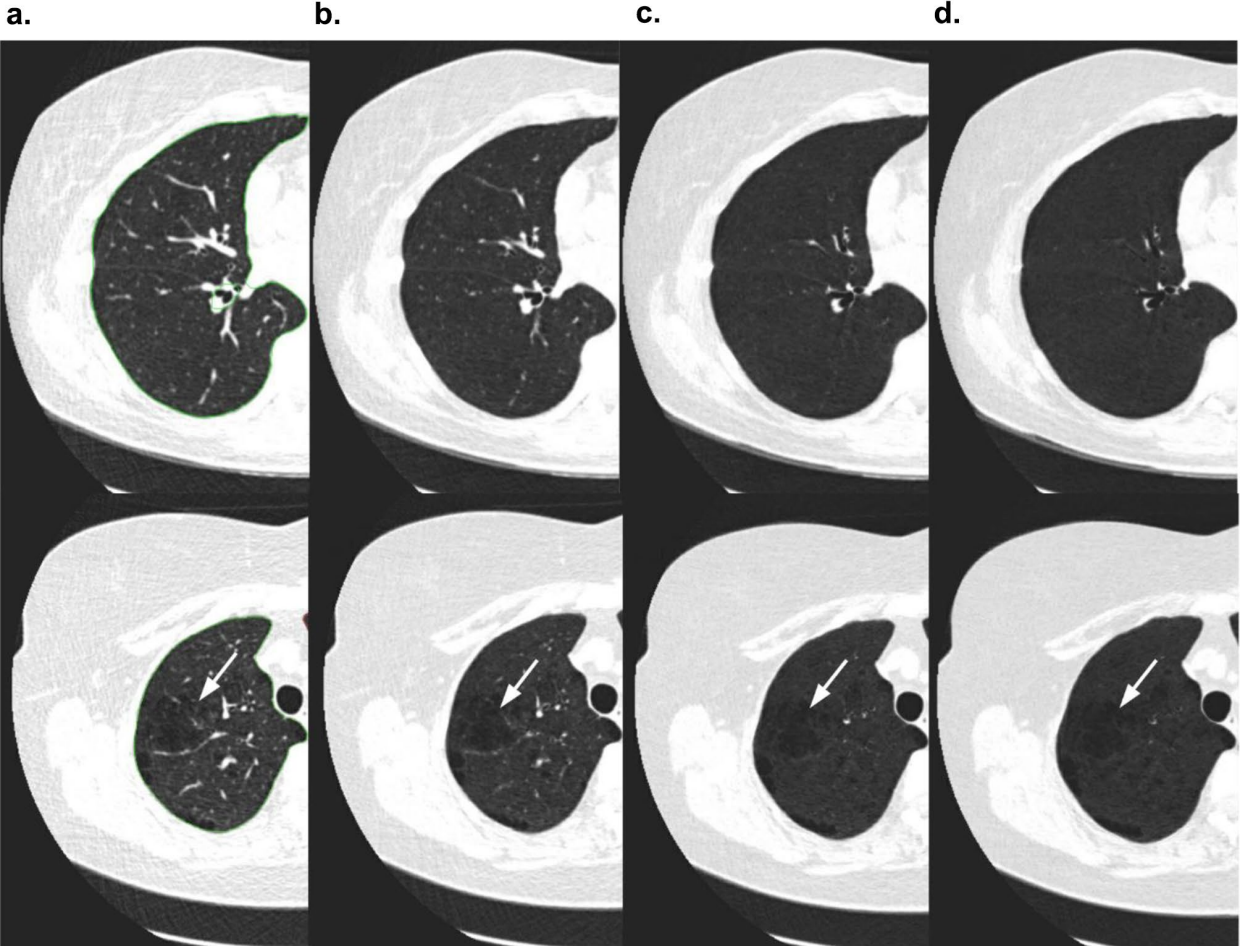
Application of various minimum intensity projection slab thicknesses on thin-section CT obtained at the same anatomic level and magnified views of the lung (window width, 500 HU; window level, -850 HU). a. Thin-section CT scan (1 mm-collimation) and b, c and d minimum intensity projection images with 3 mm, 7 mm, and 11 mm collimation. The first row represents a 52-year-old participant with non-emphysema diagnosis and the bottom row represents an emphysema participant of age 60 years. Note progressive suppression of vascular structures from 3 to 7 mm slab thickness, and better visualization low attenuation areas (white arrow) (For interpretation of the reference to color in the figure legend, the reader is referred to the web version of the article)
